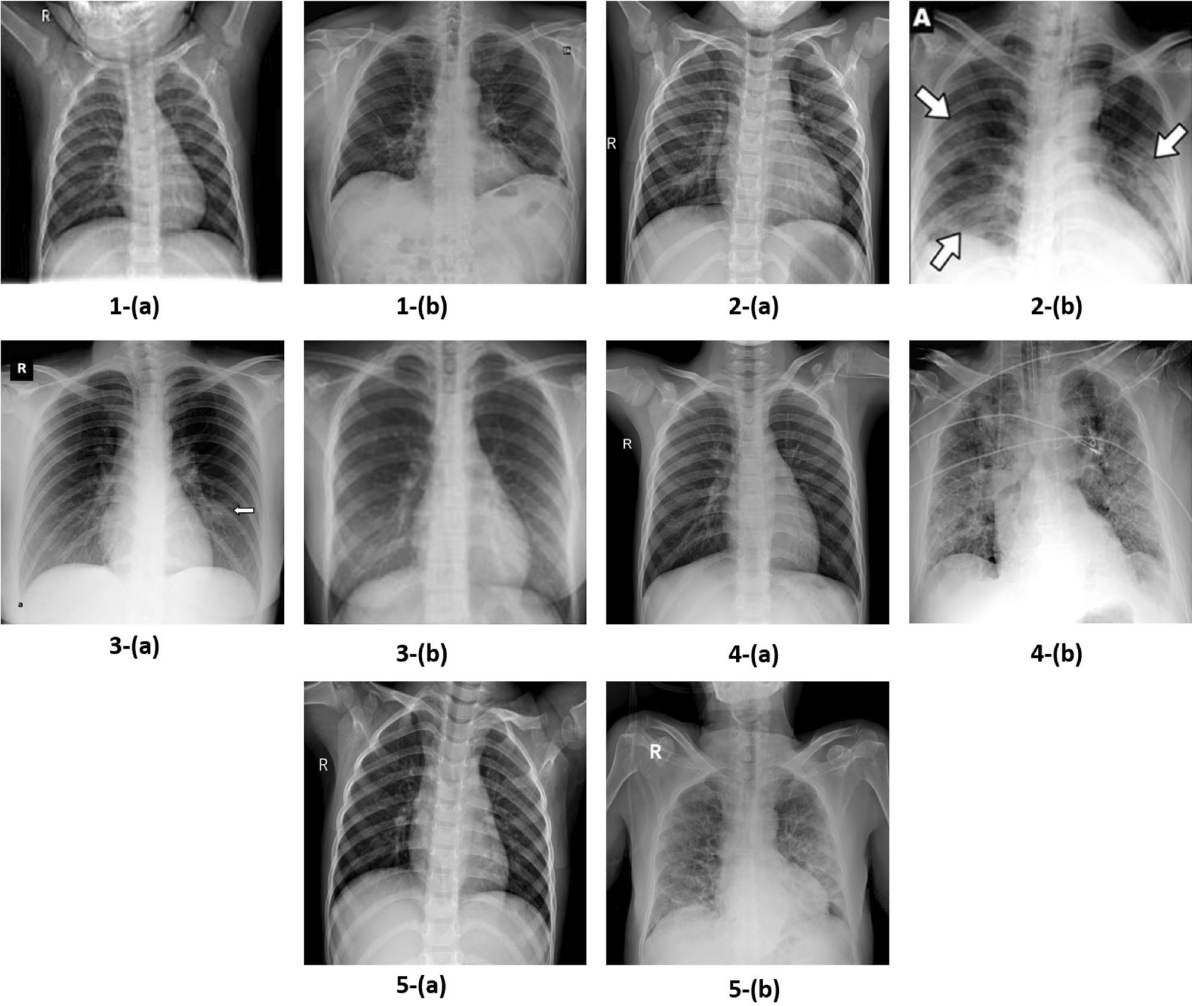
Sample images of COVIDx dataset from various repositories, where (a) represent normal image and (b) represent COVID-19 image from each repository, such as, 1. ActualMed COVID-19 CXR Dataset Initiative^33^, 2. COVID-19 radiography database^61^, 3. COVID-19 CXR Dataset Initiative^62^, 4. COVID-19 Image Data Collection^63^, and 5. COVID-19 CXR dataset^35^.
